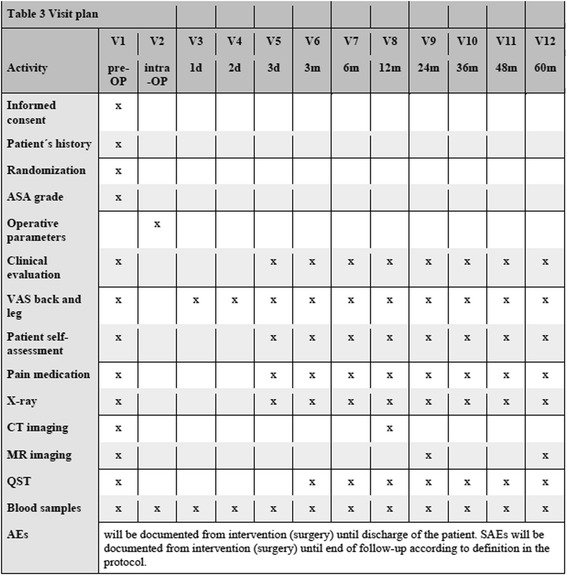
Visit plan. Patients will be followed for 5 years after the intervention. AE, adverse event; ASA, American Society of Anesthesiologists; CT, computed tomography; d, day; intra-OP, intraoperative; m, month; MR imaging, magnetic resonance imaging; QST, quantitative sensory testing; pre-OP, preoperative; SAE, severe adverse event; V, visit; VAS, visual analogue scale
